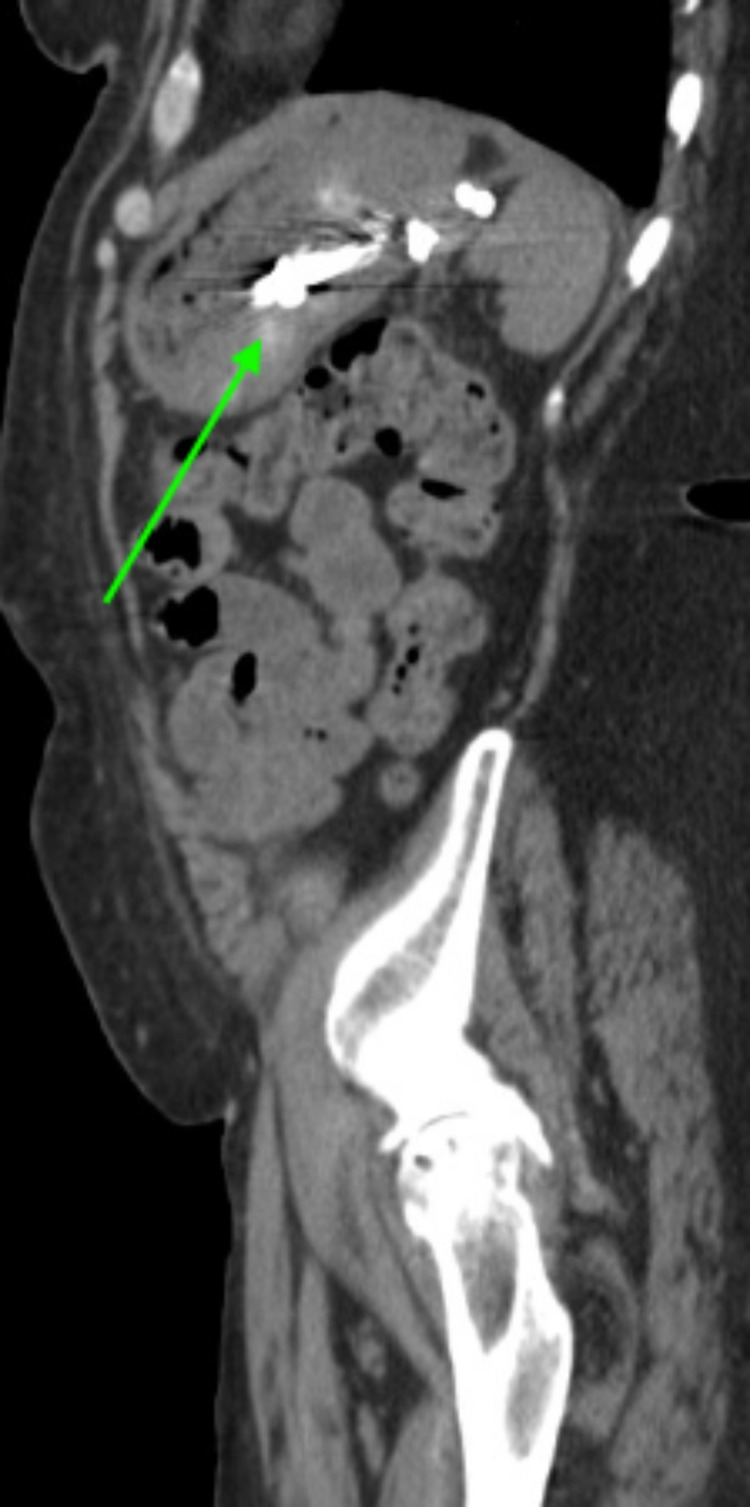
Sagittal view CT of the abdomen and pelvis with intravenous contrast at three-month follow-up showing a drainage catheter extending from the stomach to the collapsed pseudocyst within the left upper quadrant. There was no evidence of fluid re-accumulation. CT - computed tomography
